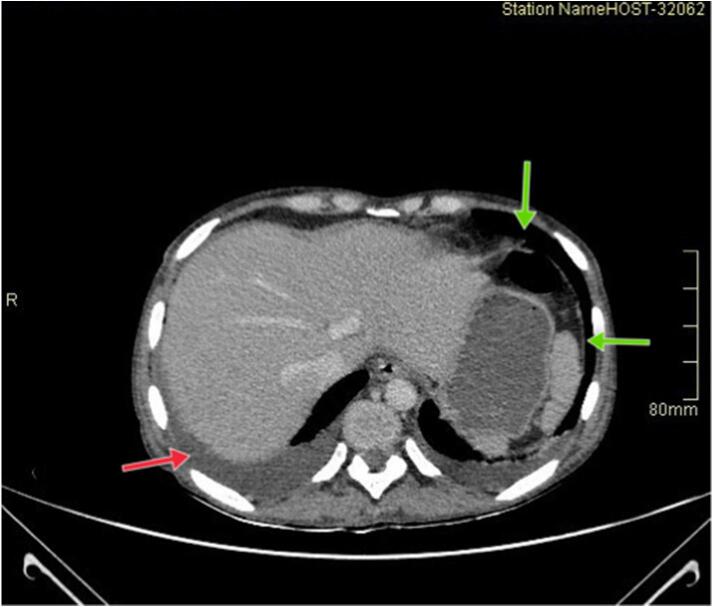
Axial CT scan image of the abdomen with significant free air in the peritoneal cavity consistent with pneumoperitoneum (green arrows), with fluid collection (red arrow). (For interpretation of the references to color in this figure legend, the reader is referred to the web version of this article.)
